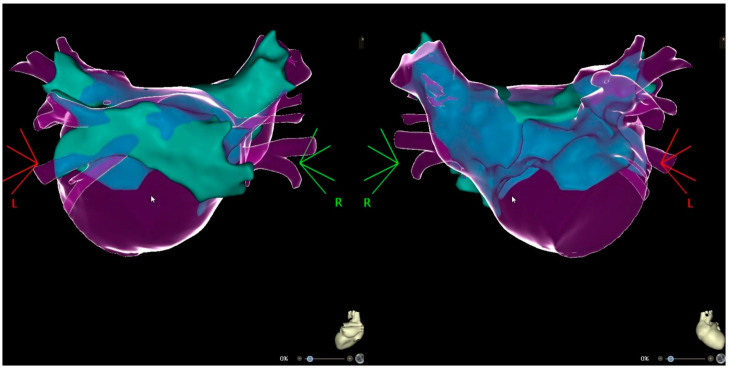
Integrated images CT scan with 3D electroanatomic map ((Left)—PA view; (Right)—AP view).
